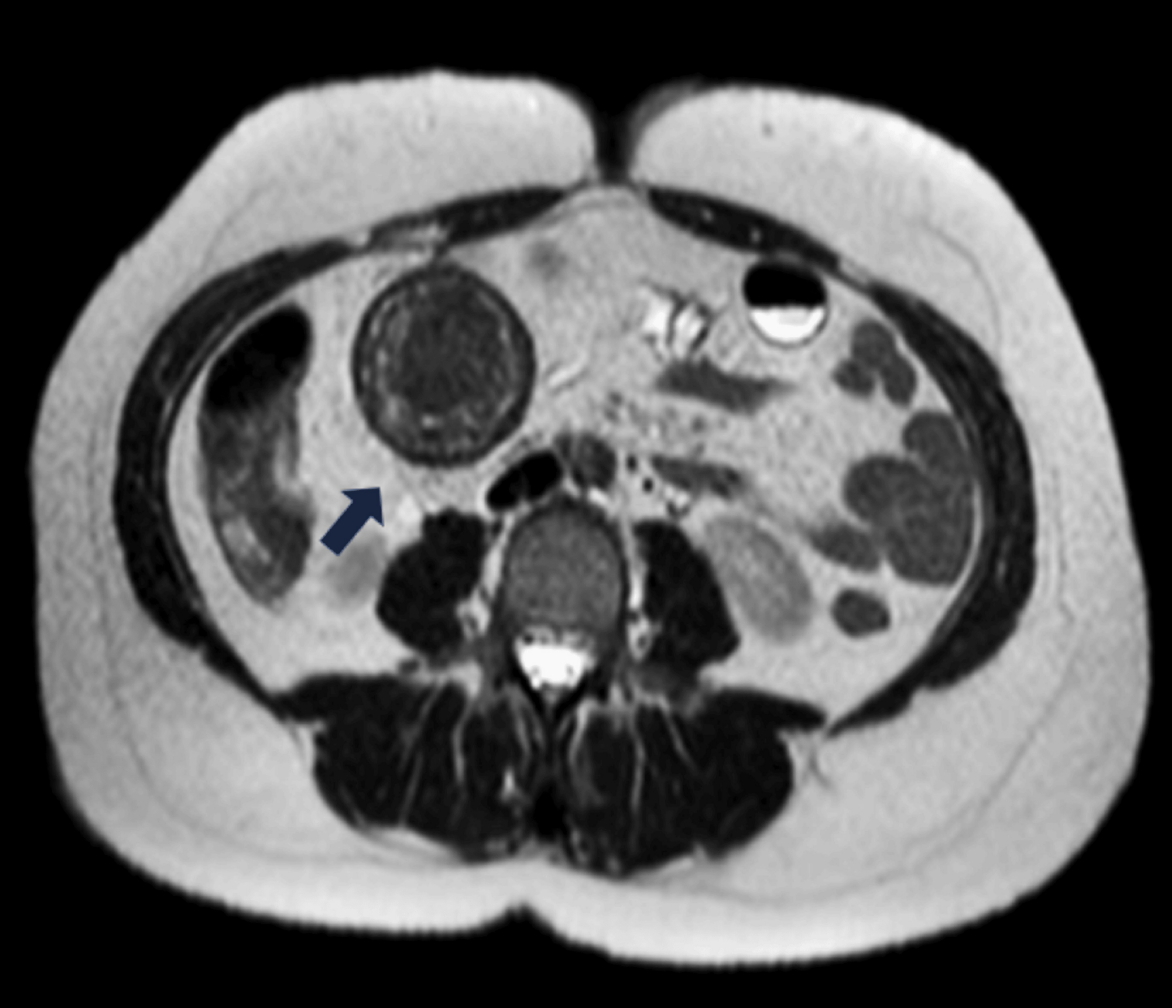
T2-weighted MRI of the abdomen revealed a distinct heterogeneous mass (black arrow) with peripheral enhancement and low signal following IV contrast administration.
